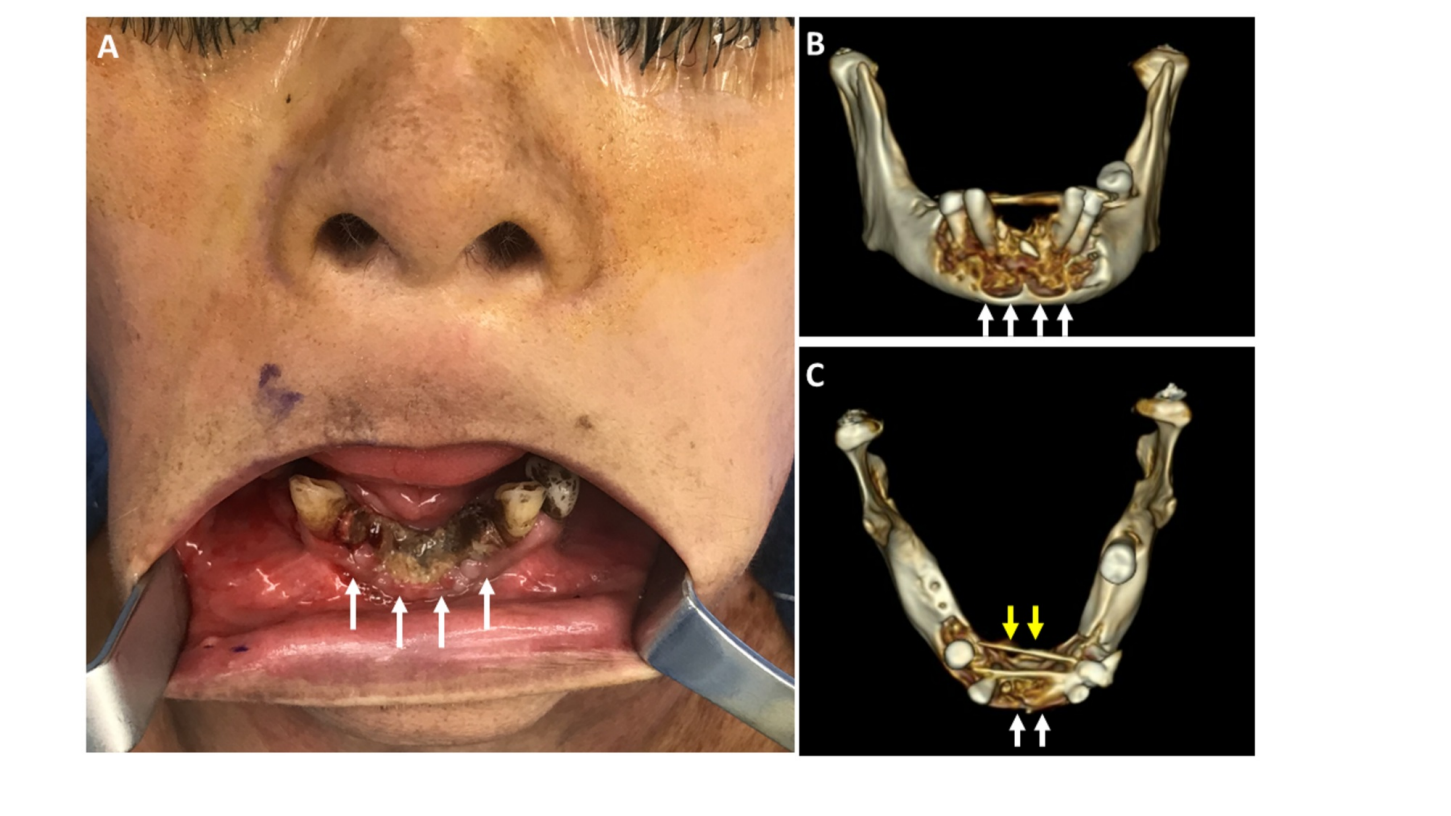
Advanced BRONJ A case of advanced BRONJ, as demonstrated by necrotic exposed mandible highlighted by white arrows (A). A three-dimensional reconstruction of the CT scan demonstrates an extensive erosive process involving the anterior mandible (B), with involvement of the facial (white arrows) and lingual cortex (yellow arrows) (C). BRONJ, bisphosphonate-related osteonecrosis of the jaw
